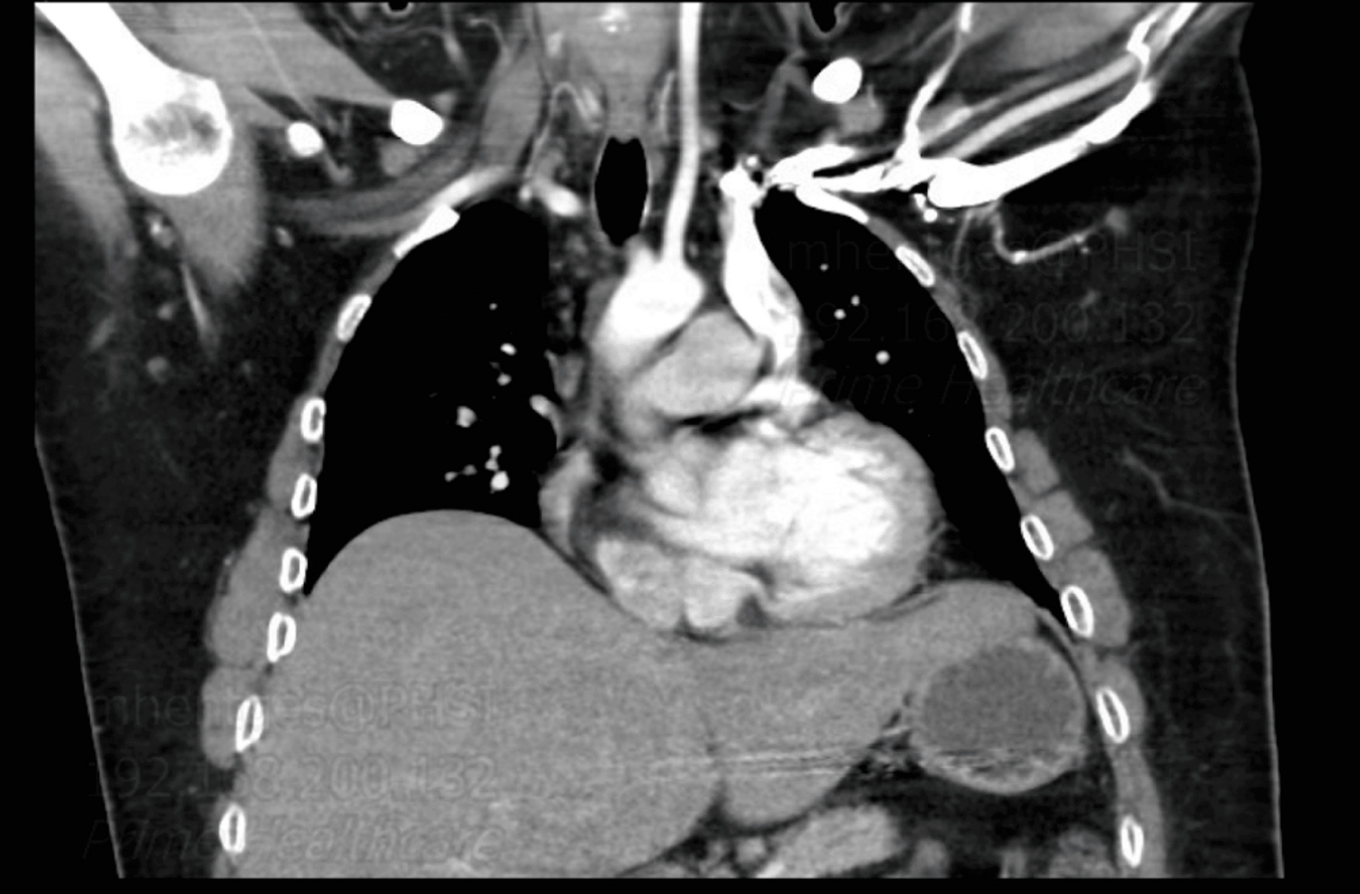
CT of the chest, 2020. Left-sided SVC dumping into the coronary sinus was noted. The aortic arch with the left common carotid can also be visualized. SVC: superior vena cava
